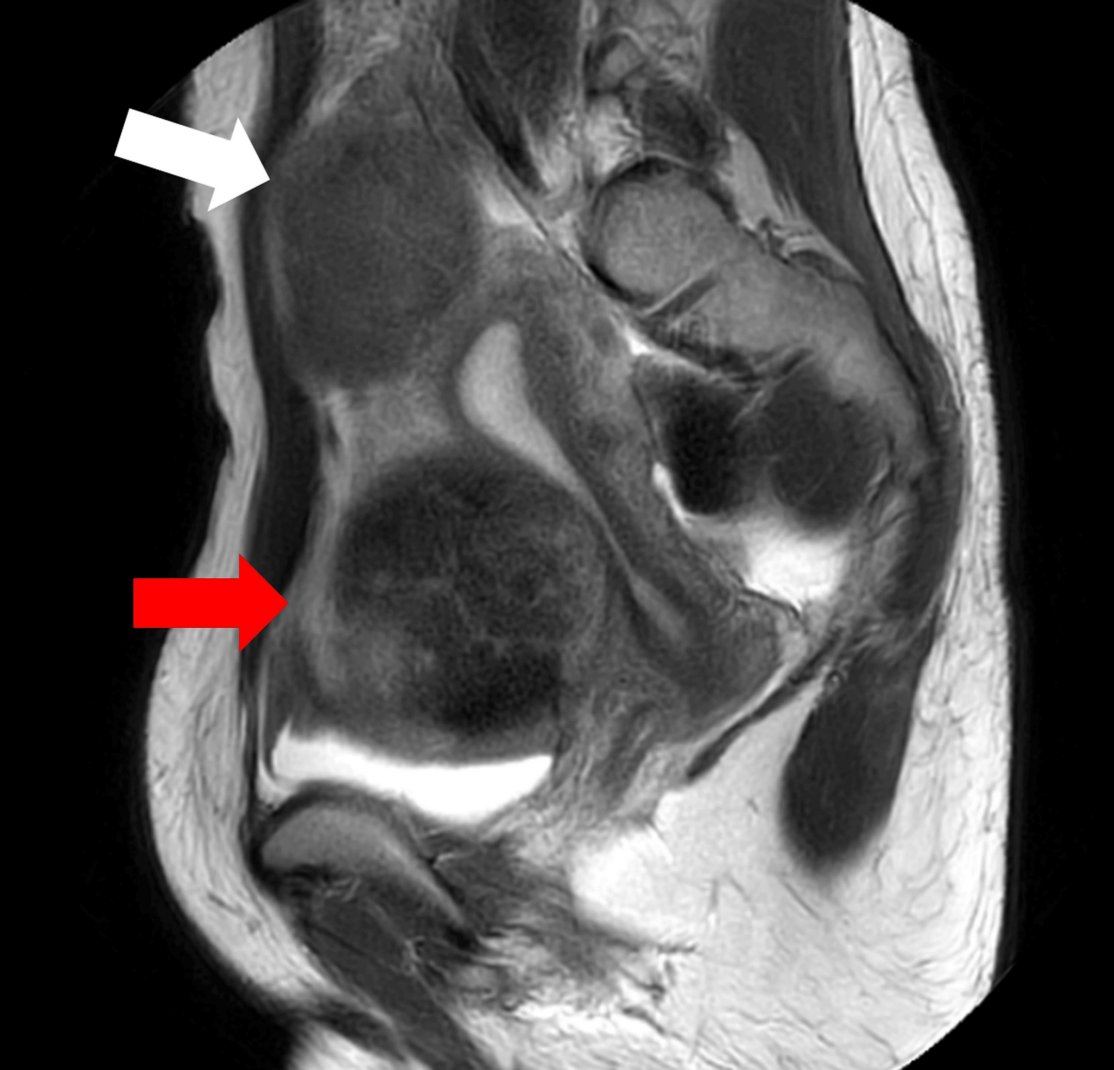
Pelvic MRI examination Pelvic MRI image showed a 6cm fibroid at the fundus (white arrow) and 9cm fibroid on the left side which distorted the uterine cavity (red arrow).
